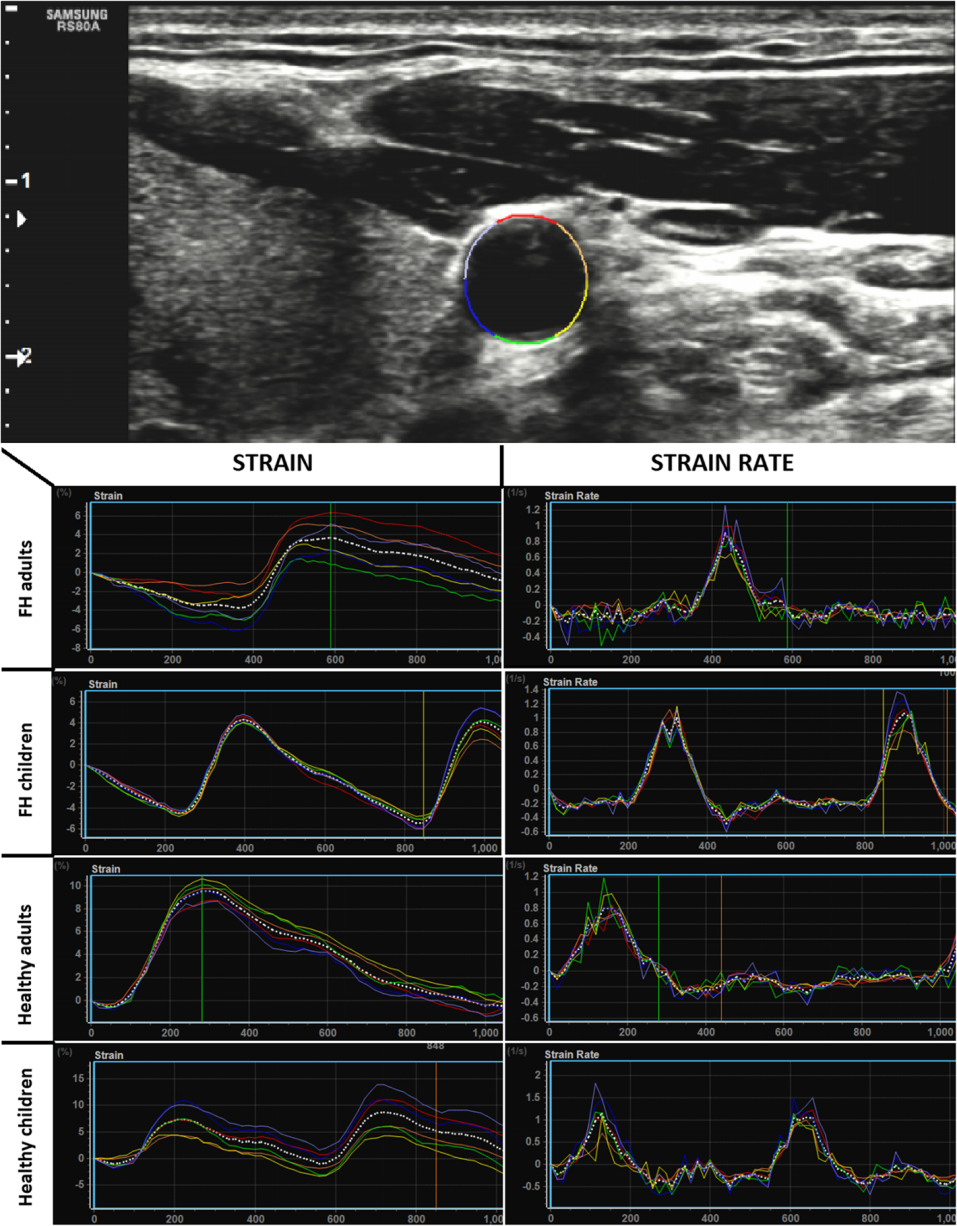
Examples of graphs presenting strain and strain rate in representative individuals from each group of patients. Colored lines represent segments of vessel while dotted white line is a mean value. At the top of the figure there is a grayscale transverse ultrasound of the neck with ROI in the CCA
